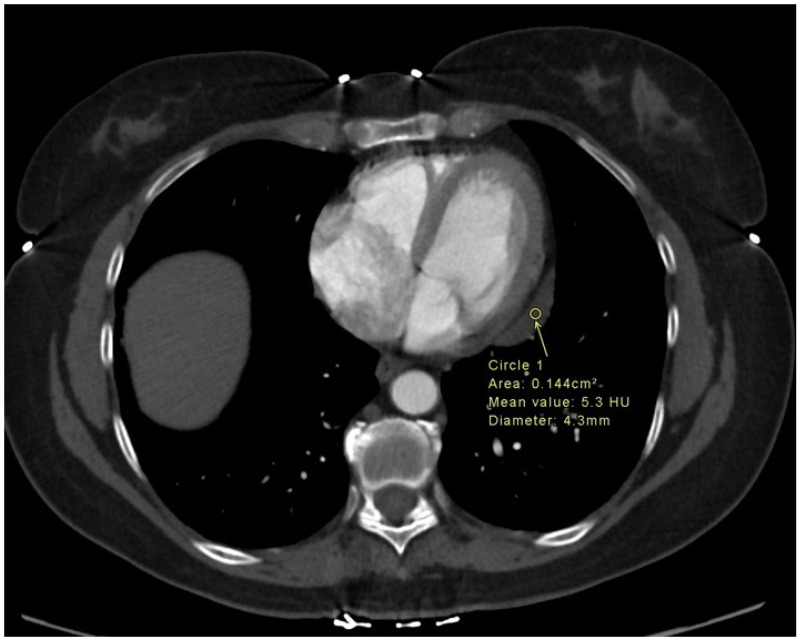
Axial computed tomography chest images depicting an incidentally detected well-defined hypoattenuating cystic lesion (yellow arrow) along the basal-mid lateral left ventricular wall consistent with a pericardial cyst.
